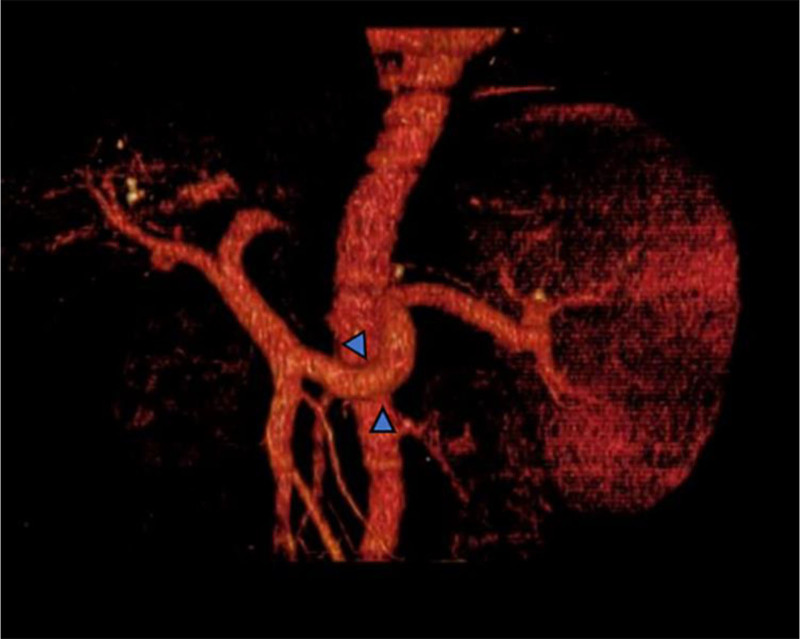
A portal vein in CTA showed portal vein hypertension and splenic portal vein varicose. CTA = computed tomography angiography.
